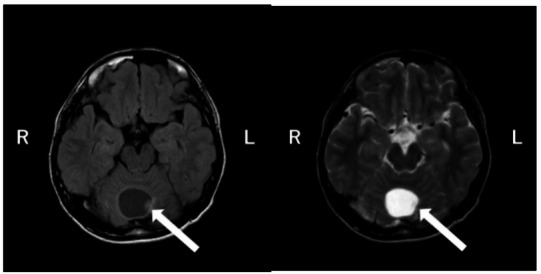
T1- and T2-weighted MRI images. T1- and T2-weighted brain MRI images show a well-circumscribed mass, approximately 3 cm in size, located in the cerebellar vermis. No other remarkable abnormalities were observed in the brain parenchyma or sulci
